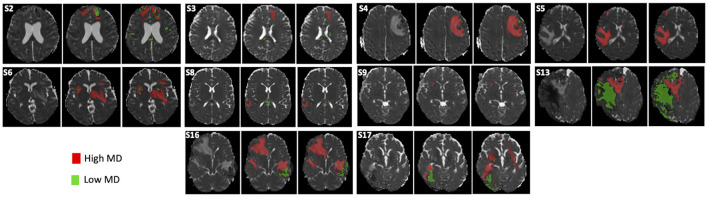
Delineation of brain lesions from diffusion-weighted images (DWI) in 10 TBI patients. The MD map (left), rater consensus (middle) and automated quantification procedure (right) is shown for each patient. S2–S17 refer to the corresponding TBI subject (see Table 3).
